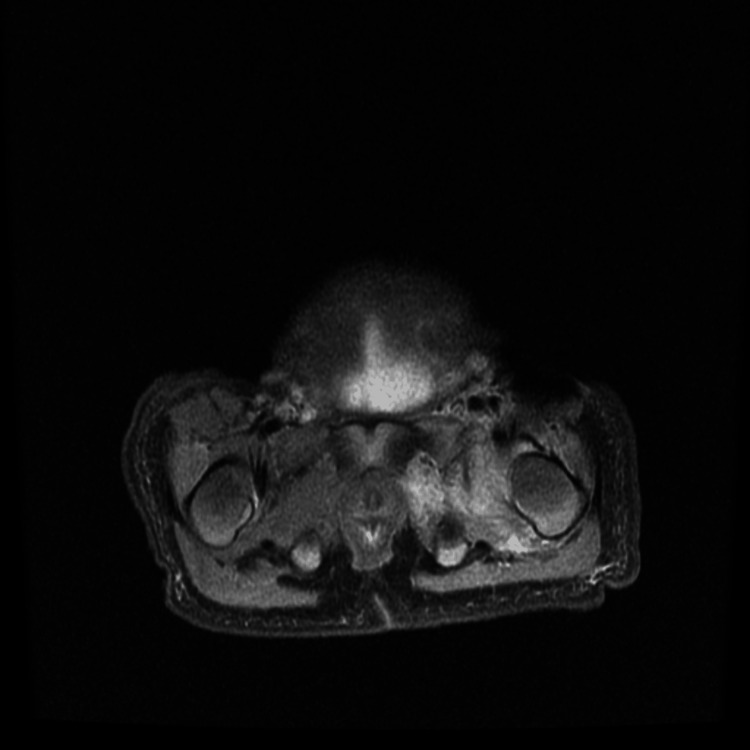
Axial T2-weighted MRI of the left hip. Axial image showing joint effusion in the left hip joint and inflammation of the surrounding soft tissues.
